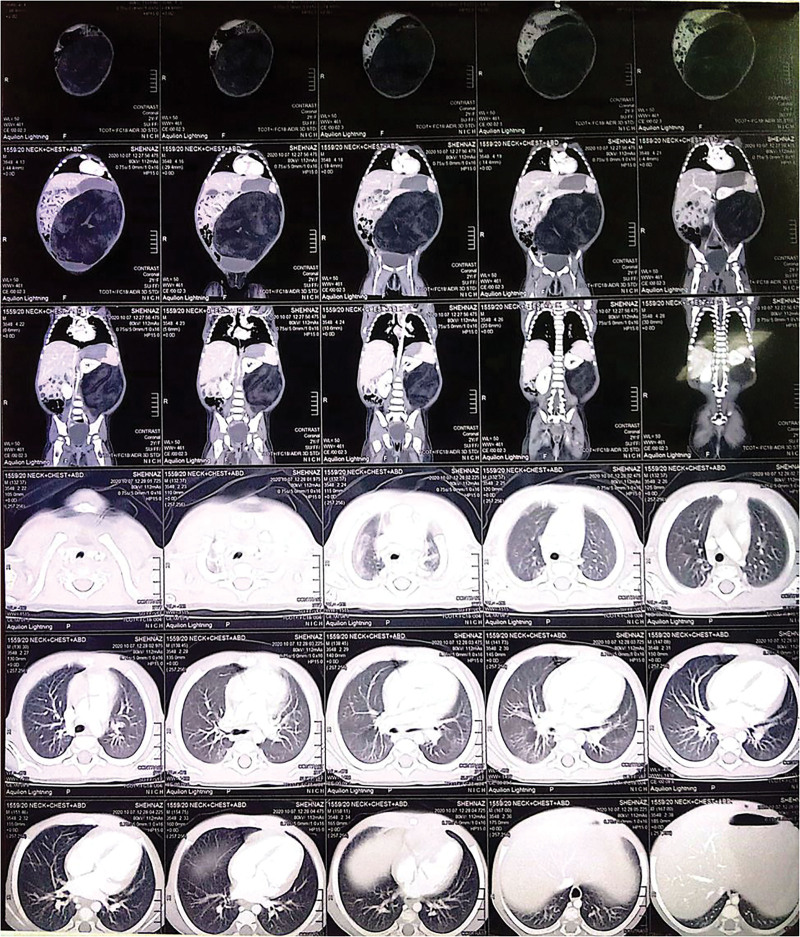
Computed tomography scan of the head, chest, and abdomen showing the retroperitoneal lipoma pushing the left kidney upwards while shifting the intestinal loops towards the right.
